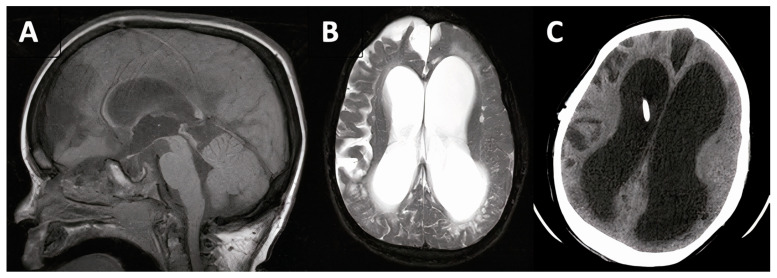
10-year-old male patient with Hunter syndrome and hydrocephalus (case #8). Pre-operative T1-weighted mid-sagittal and T2-weighted axial magnetic resonance imaging (MRI, A,B) and 1 year post-operative computed tomography scan with ventricular catheter visualized in the dilated right lateral ventricle (C).
